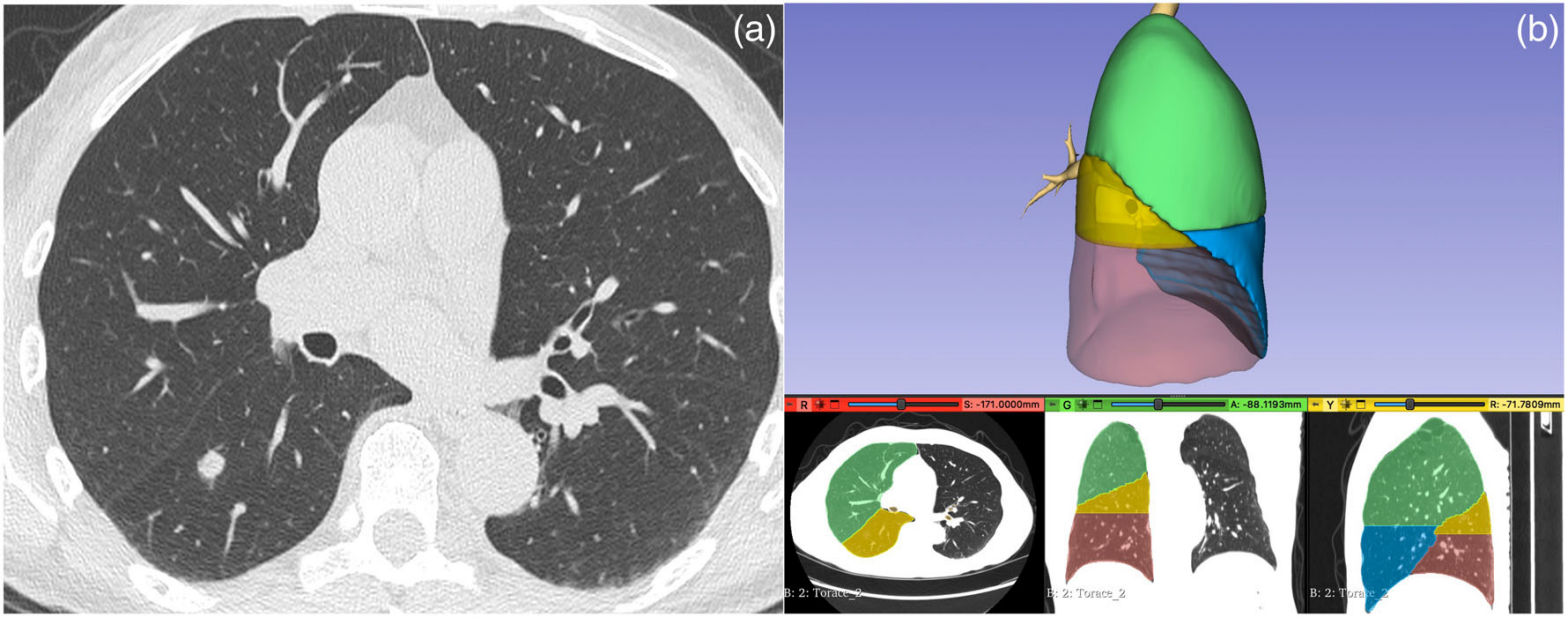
A 61‐year‐old man with a 9 mm adenocarcinoma. (a) Computed tomography (CT) scan showed tumor located in right S6. (b) Digital 3D model with right S6 (yellow) and right lower lobe (RLL) in transparency (red), middle lobe (ML) (azure), right upper lobe (RUL) (green) and nodule (black) in S6.
